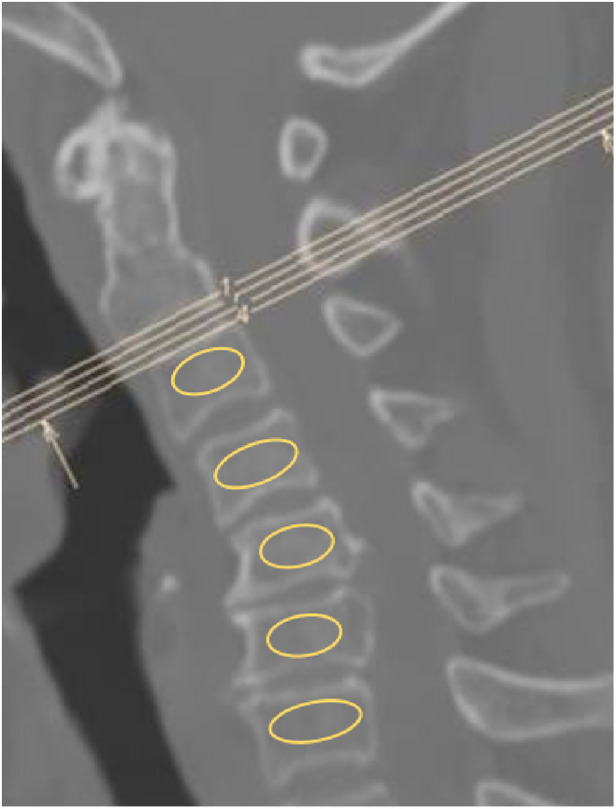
Using an elliptical region of interest function to evaluate the hounsfield units (HU) value in median sagittal computed tomography (CT) scan of the cervical spine, select the largest possible range of cancellous bone without including cortical bone.
